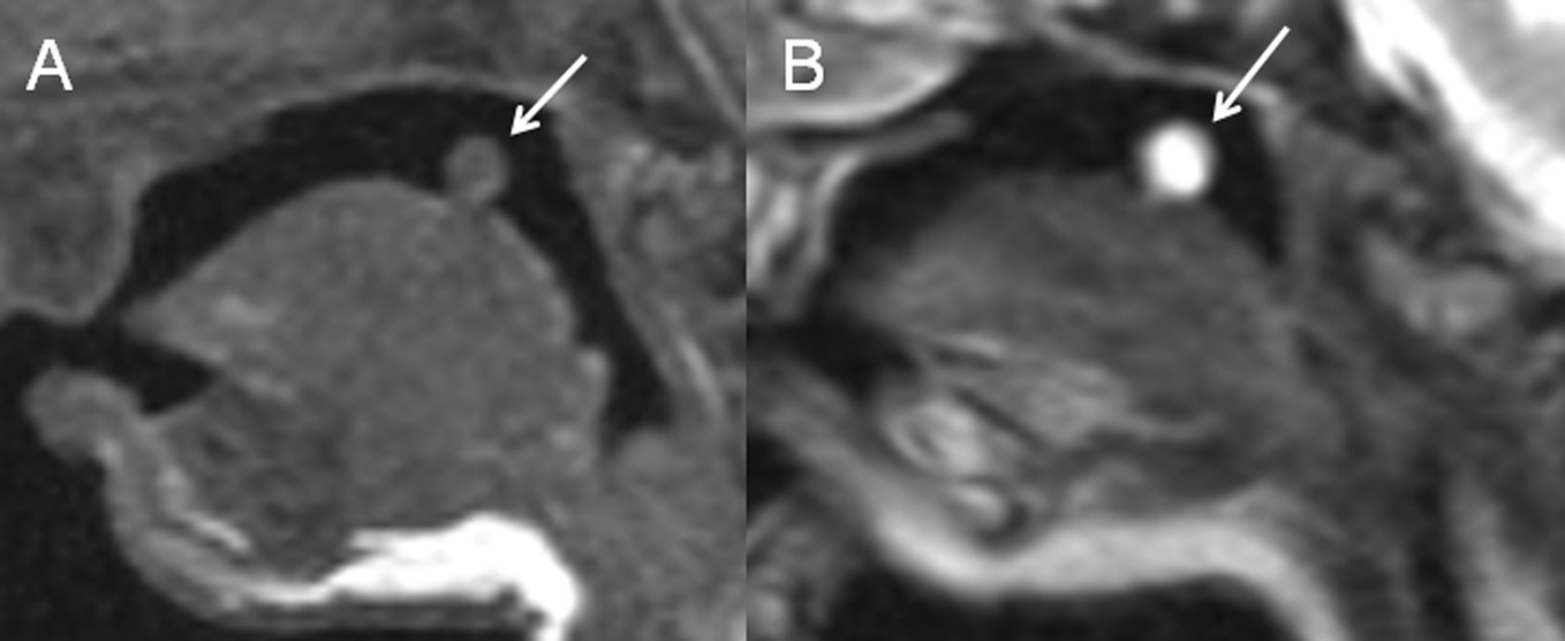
A, sagittal T1-weighted and B, sagittal T2-weighted MR image of a 2-week-old newborn with OFD VI demonstrate a T1-isointense (white arrow on A) and T2-hyperintense (white arrow on B) tissue lesion on the surface of the posterior part of the tongue, representing a tongue hamartoma.
